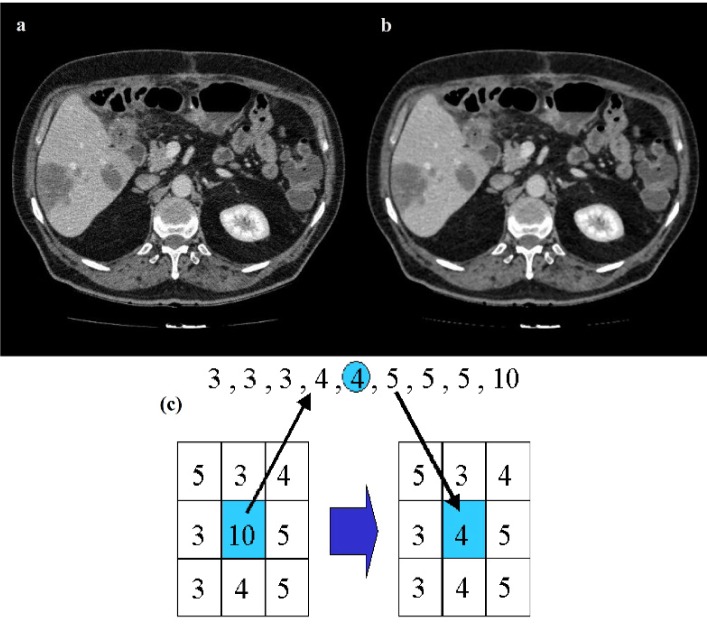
Results of median filtering, original CT image (a), filtered CT image (b), median filter (c)
